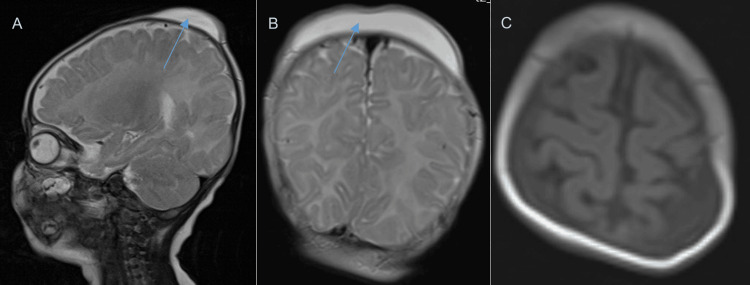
Sagittal (A) and Coronal (B) T2-weighted MRI scan revealing a well-defined hyperintense (fluid-filled) collection external to the skull in the subaponeurotic space.; Axial MRI scan (C) showing a localized hypointense scalp.
